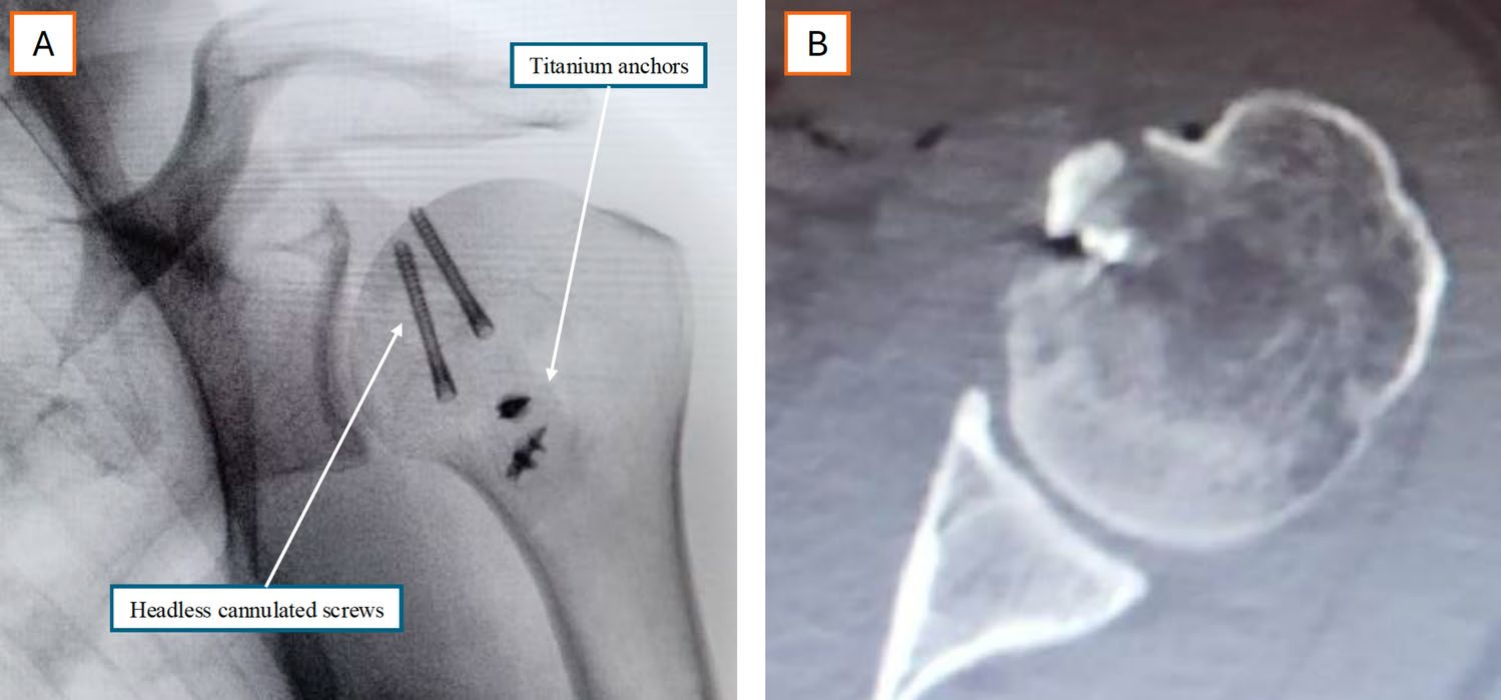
Postoperative imaging. A Anteroposterior radiograph showing headless cannulated screws and titanium anchors for tuberosity fixation. B Axial CT scan confirming restoration of the articular surface. Orientation: right shoulder
